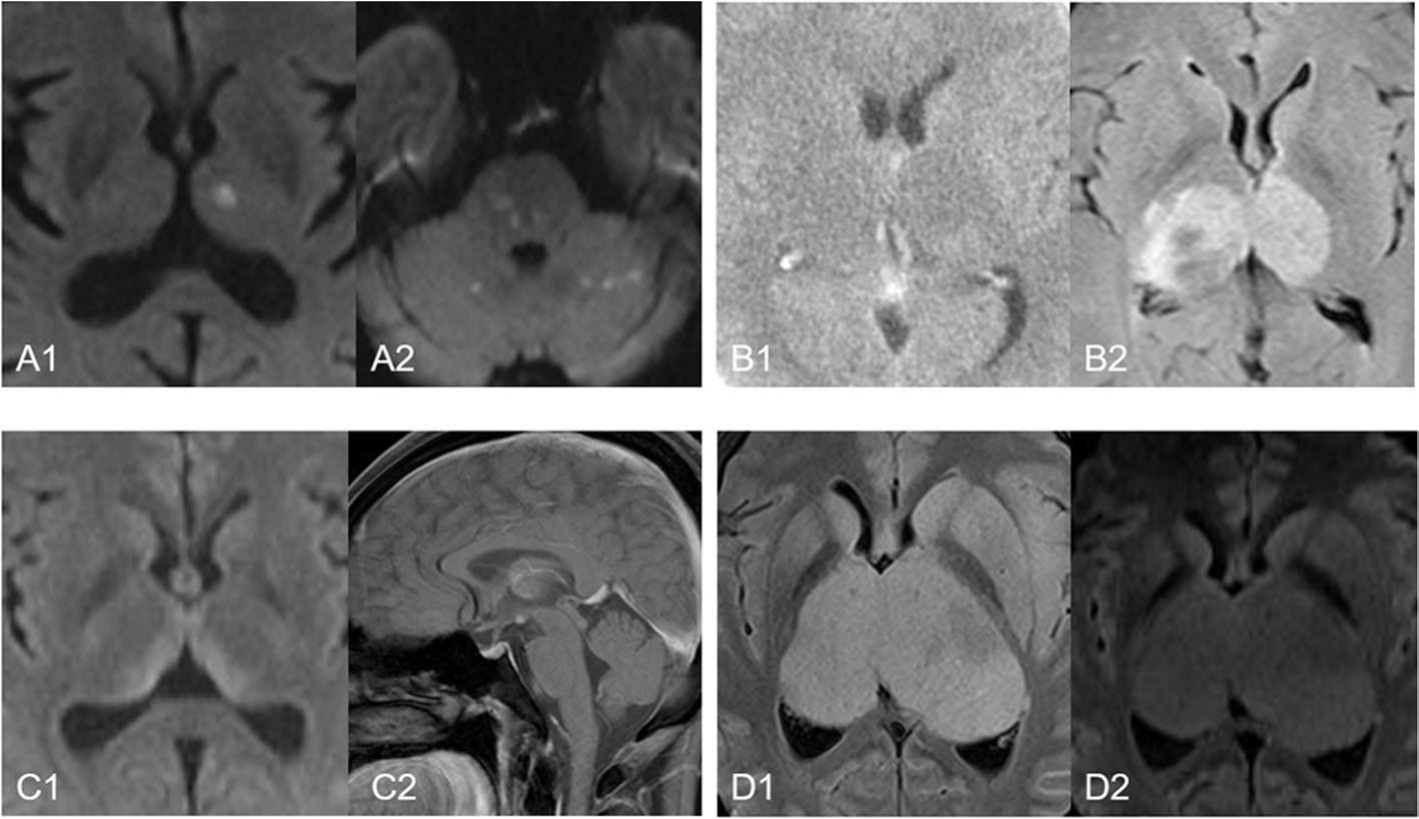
Differential diagnosis of AOP infarction. Top of the basilar artery syndrome can lead not only to thalamic infarction, but also to additional characteristic cerebellar, brainstem and occipital lobe infarction (A1 and A2). For deep vein infarction caused by thrombosis, high density of bilateral internal cerebral veins (B1, black arrow) can be observed on head CT, and FLAIR shows angiogenic edema involving multiple arterial areas of thalamus (B2). Wernicke’s encephalopathy often implicates pulvinar (C1) with abnormal enhancement of papillary body (C2, white arrow). Diffuse midline glioma in bilateral thalamus with mass effect is hyperintense on FLAIR and isointensity on DWI (D1 and D2)
